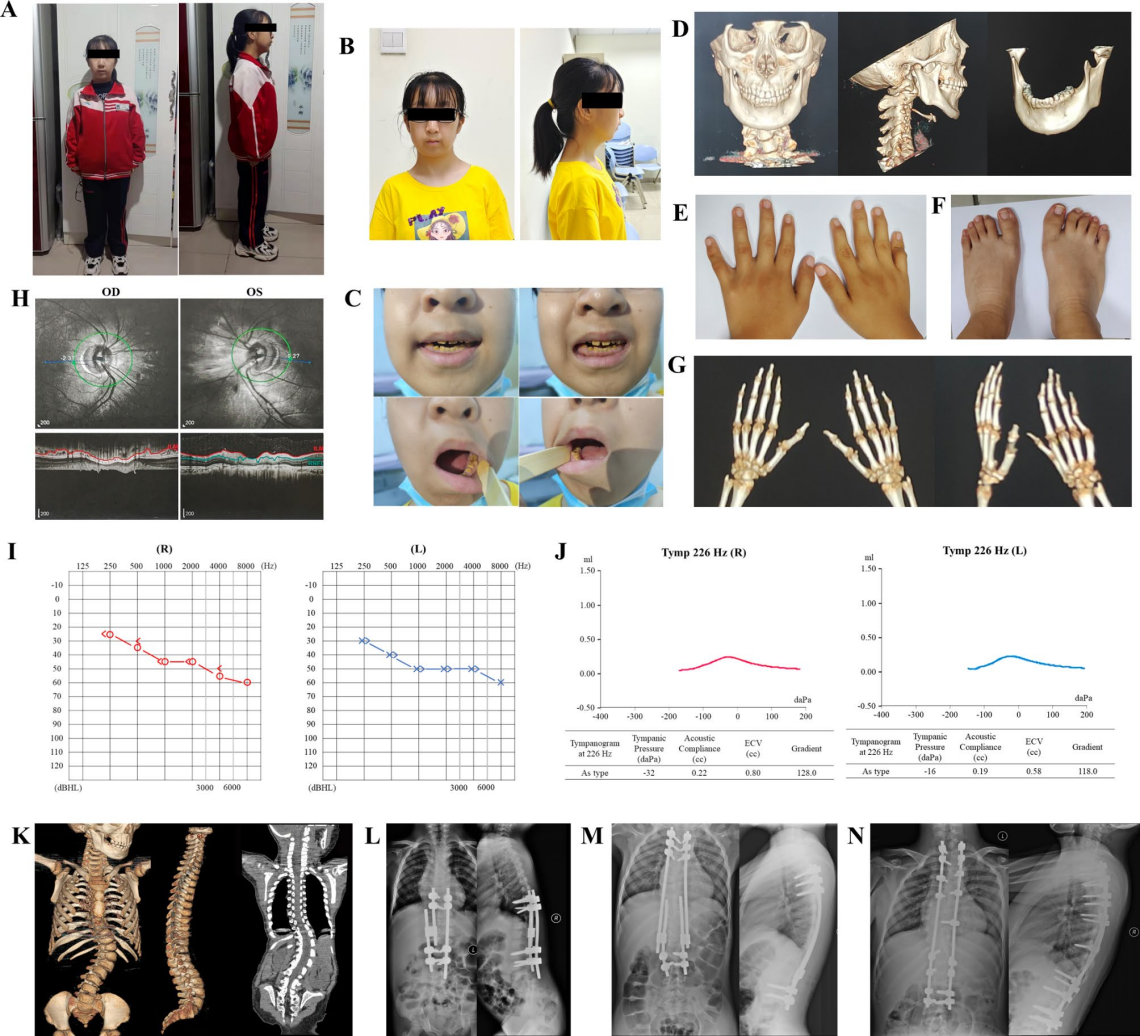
Clinical features of patient. (A) Short stature. (B) Facial dysmorphism including flat face. (C) Hypomineralised amelogenesis imperfecta. (D) CT of skull showing flat face and amelogenesis imperfecta. (E) Brachydactyly of patient’s hands. (F) Mild flat feet. (G) CT of hands showed brachydactyly and contracture of the little finger joint. (H) Optical Coherence Tomography of patient showed light optic disc color in both eyes, increased the cup-to-disc ratio, optic nerve atrophy and thin optic nerve atrophy. (I) Moderate hearing impairment. (J) As type tympanogram of patient at 226 Hz of both ears. (K) CT images of patient before surgery when 3 years old. Obvious scoliosis was observed at thoracic and lumbar vertebrae. (L) The X-ray images of patient after her first single-side growth rod surgery. Pedicle screws were fixed at T9, T10, L3 and L4. (M) The X-ray images of patient after surgical replacement of the single-side growth rod by dual growth rod at the age of 10.2 years. Screws were fixed at T1, T2, T3, T5, T8, T11, T12, L2 and L3 at the left side and T1, T2, T4, T5, T10, T12, L2 and L3 at the right side. (N) X-ray images of patient after her final fusion surgery. Old screws were removed and a new set of screws was fixed at previous vertebral bodies. Abbreviations, R, right; L, left; OD, Oculus Dexter; OS, Oculus Sinister; ILM, internal limiting membrane; RNFL, retinal nerve fiber layer. T, thoracic; L, lumbar
